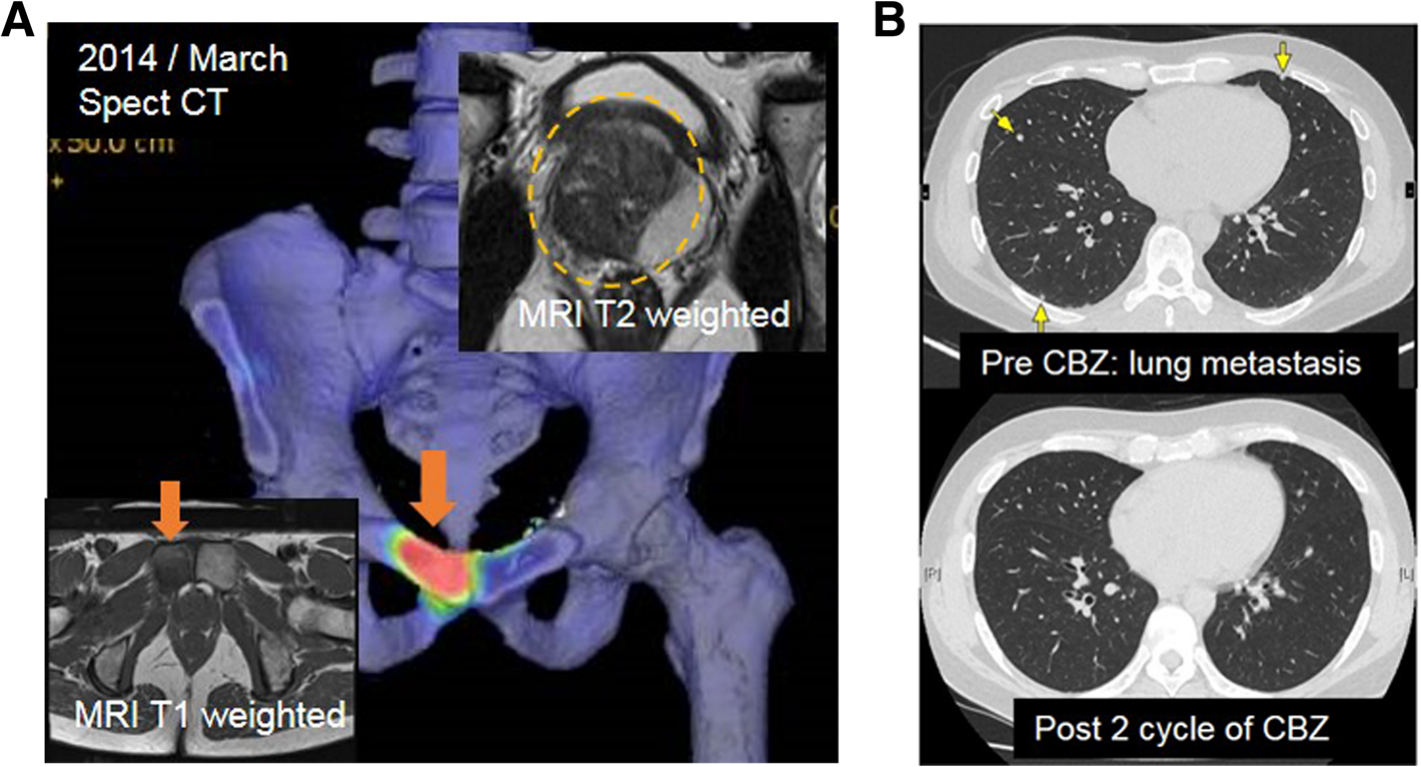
a Magnetic resonance imaging and bone single-photon emission computerized tomography show a prostate tumor invading the seminal vesicle and bone metastasis in the pubic bone. b Positron emission tomography-computed tomography shows tiny nodules in the bilateral lungs. Following 2 cycles of cabazitaxel chemotherapy, the lung metastases completely disappeared
